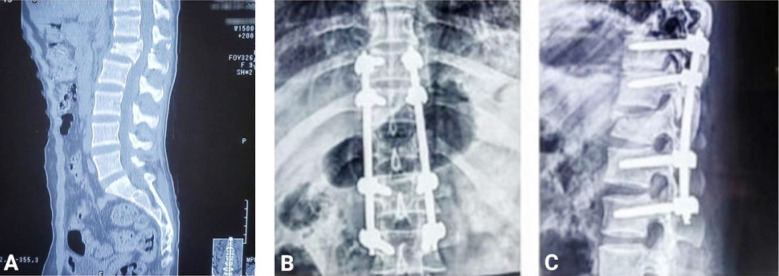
A: Preoperative CT TL spine showing L1 fracture, B&C: Postoperative X-rays AP and lateral views for LSPF.
